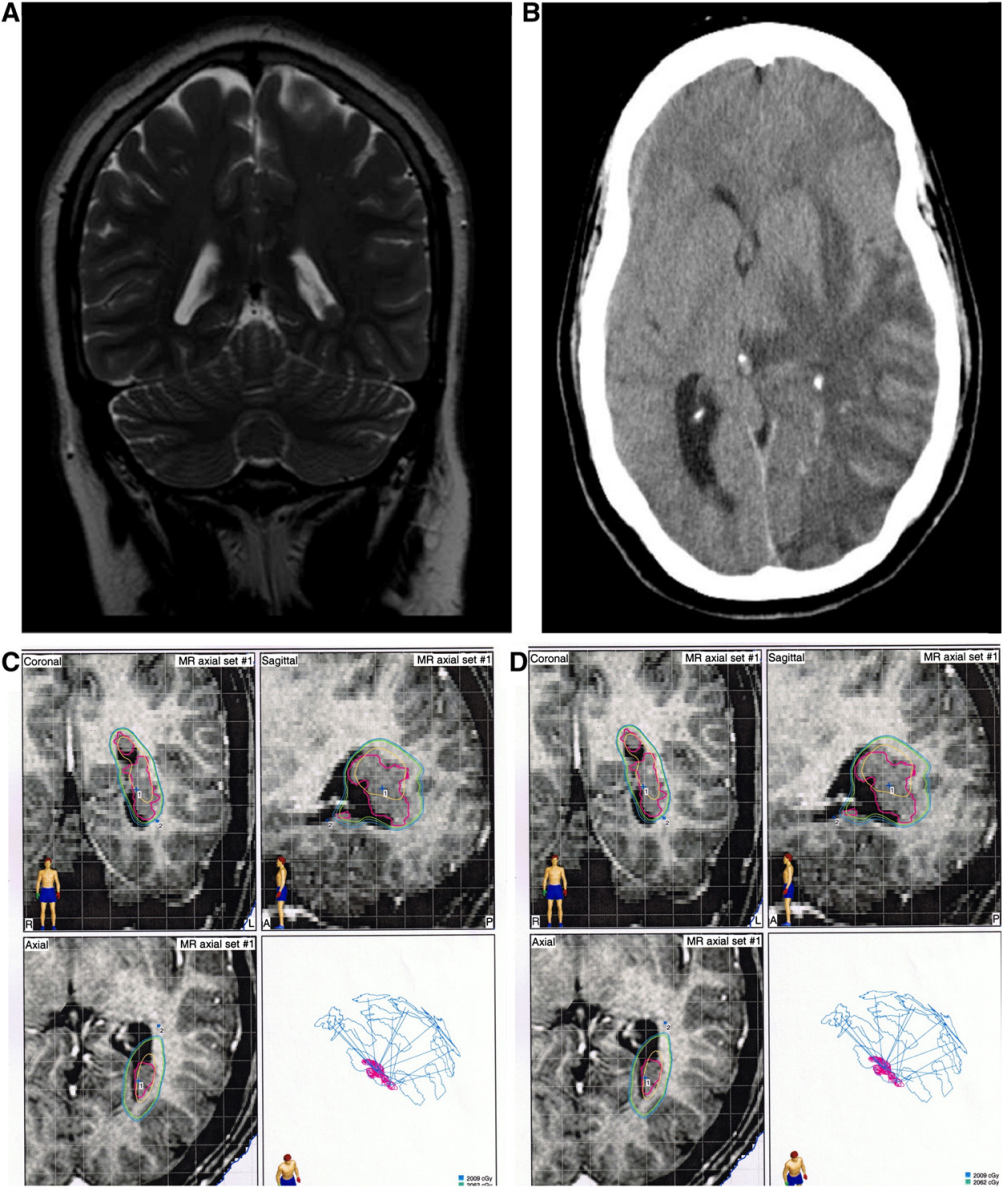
A: Coronal T2 MRI showing left trigone PVH. B: CT scan demonstrating cerebral edema from radiation necrosis. C: Dose plan for initial LINAC treatment for Case 2. D: Dose plan for second LINAC treatment for Case 2.
